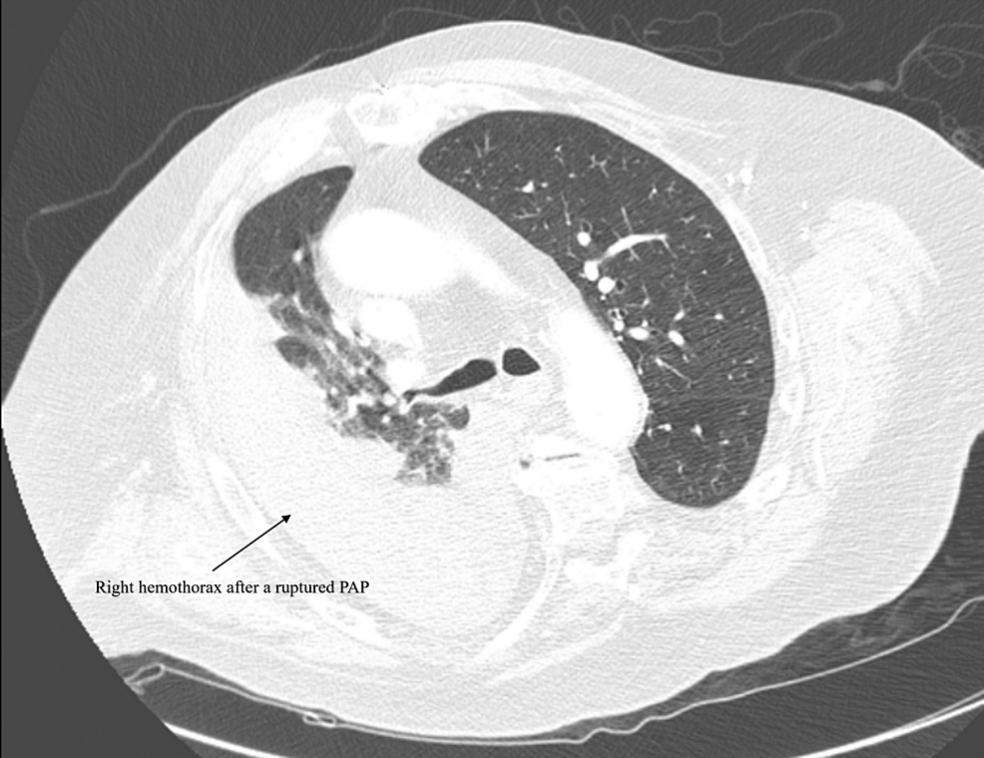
CT chest showing large hematoma in the right pleural space PAP, pulmonary artery pseudoaneurysm
